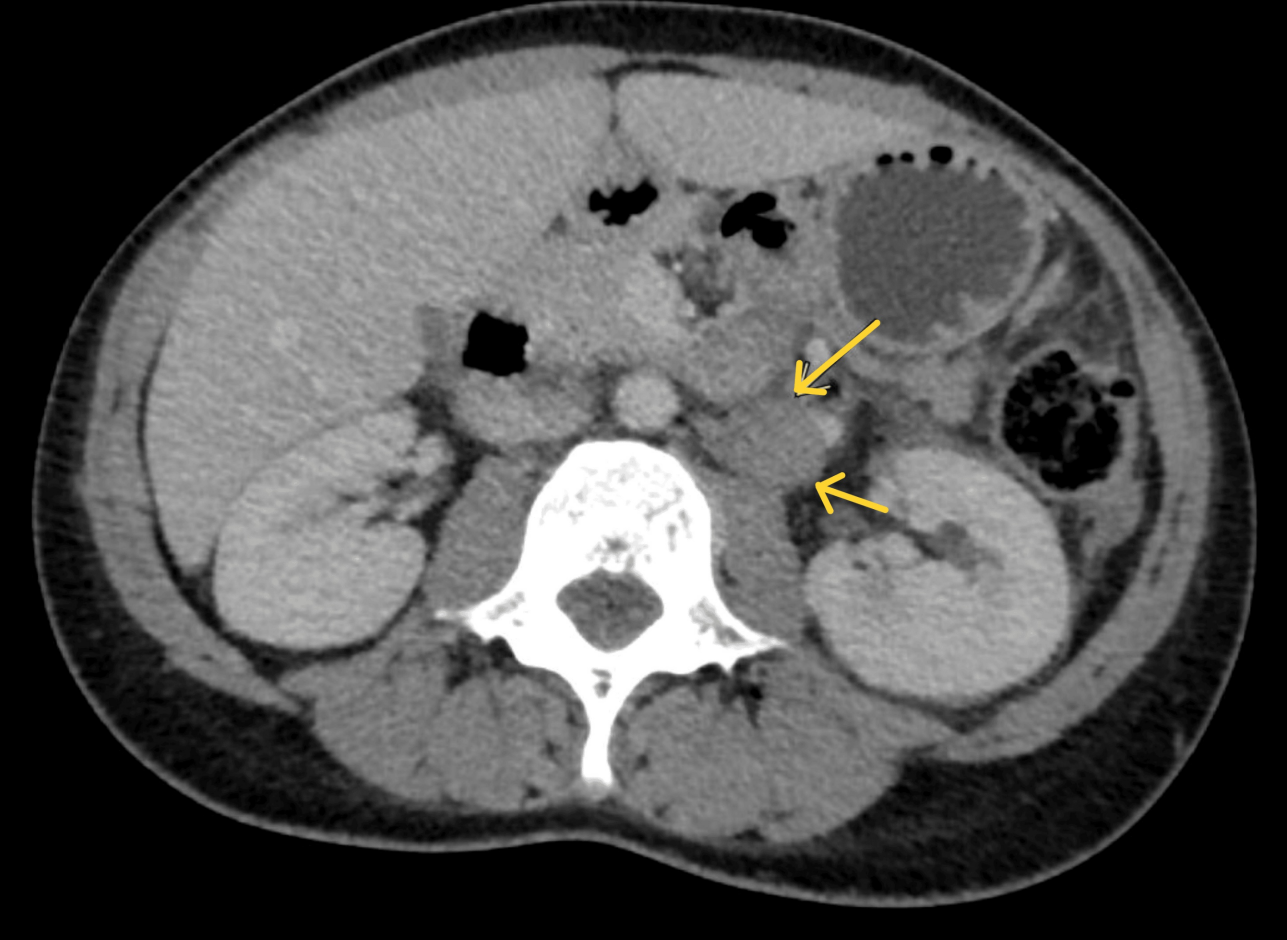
Axial computerized tomography (CT) scan of the abdomen The arrows indicate left paraaortic lymph node enlargement.
